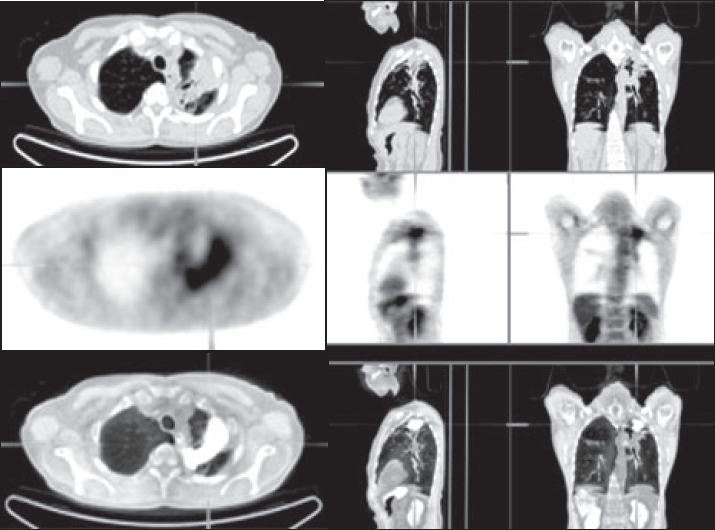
Non-small cell lung cancer. PET/CT images show invading the visceral pleura without chest wall invasion while on the CT alone it would be more difficult to determine the chest wall invasion (T2 N0 M0) (Reprinted with permission of reference).[39]
